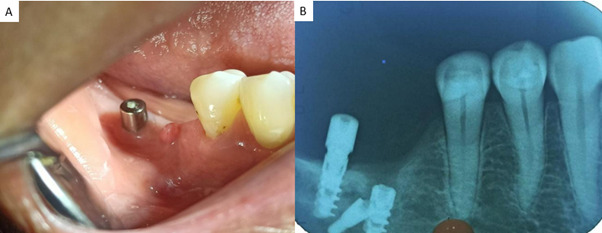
A) Sector posterior derecho mandibular con el pilar del implante dental correspondiente a la pieza 4.7. B) Radiografía periapical donde se observa la presencia de tres implantes dentales.
